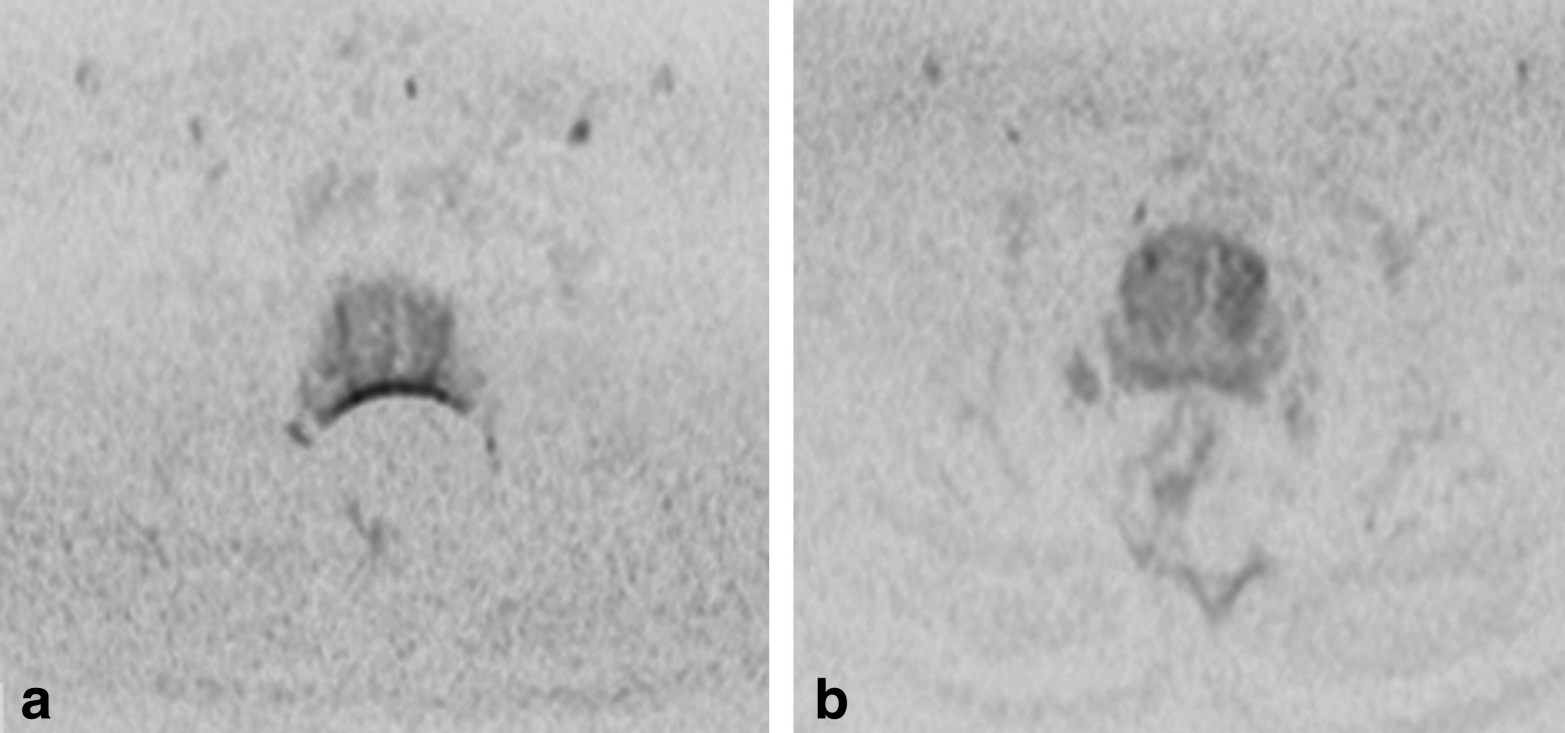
Prostate imaging. DWI b-800 demonstrates marked susceptibility from gas in the rectum (a) obscuring visualization of the peripheral zone. This can be simply rectified noninvasively by prone positioning (b) of the patient, or with rectal gel.
